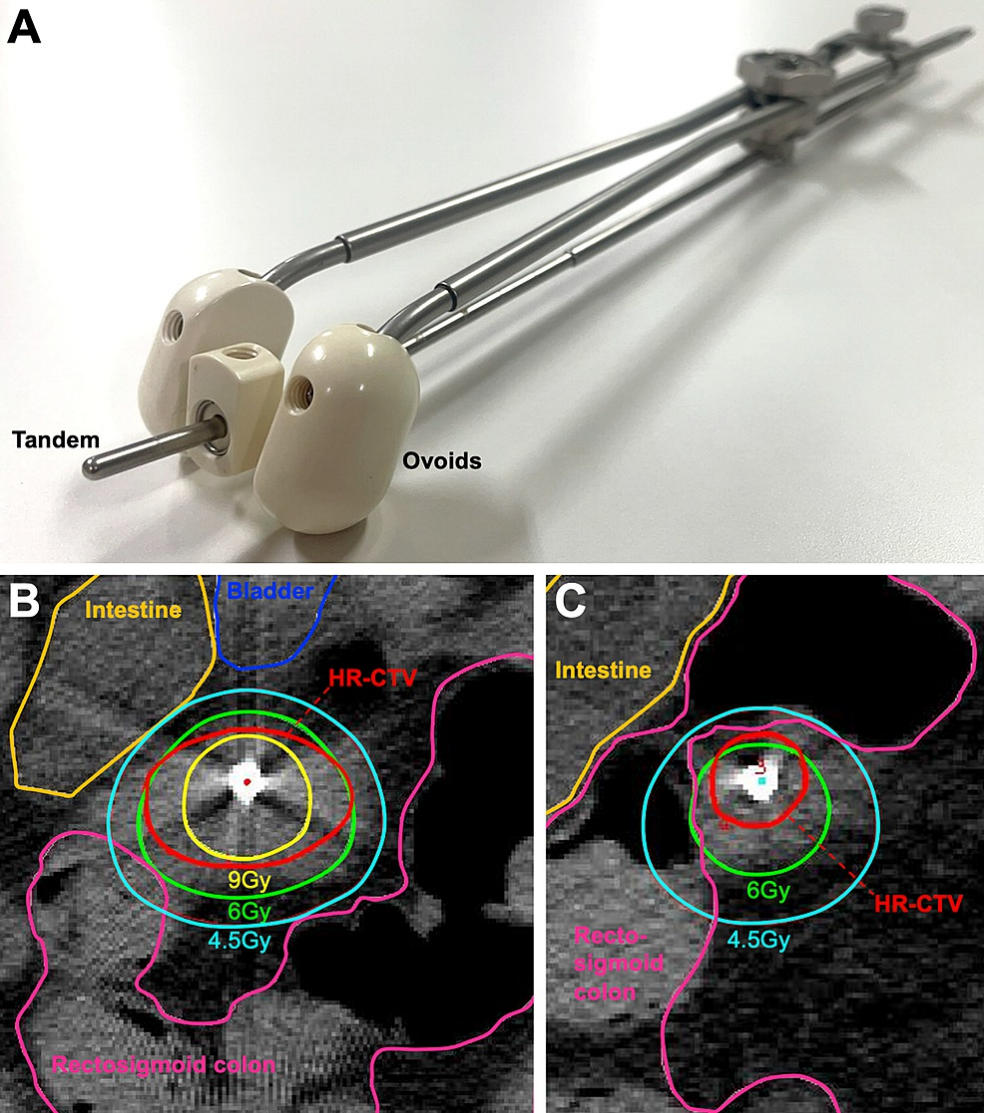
Brachytherapy settings. (A) The tandem (2 cm in depth) and ovoid applicators. (B, C) Representative images of computed tomography-based brachytherapy planning. (B) Axial plane in which the high risk-clinical target volume (HR-CTV, red) shows the maximal diameter. (C) Axial plane showing the cranial end of the HR-CTV. Note that the contours of HR-CTV are broadly covered by the iso-dose lines denoting 6 Gy (green), whereas those for the rectosigmoid colon (magenta) surrounding HR-CTV are not.
